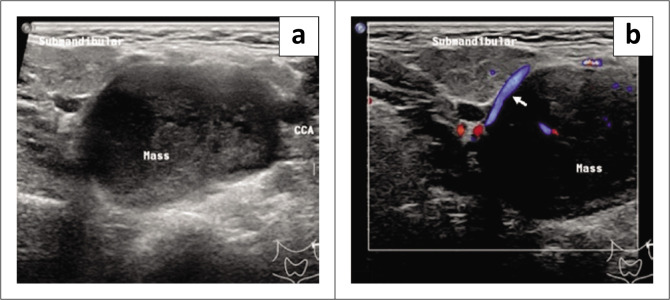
(a) Transverse greyscale ultrasound of the neck revealed a well-defined hypoechoic mass lateral and inferior to the left submandibular gland and medial to the left common carotid artery. (b) Transverse Doppler ultrasound of neck. Note minimal internal vascularity (white arrow) demonstrated on colour Doppler ultrasound.
